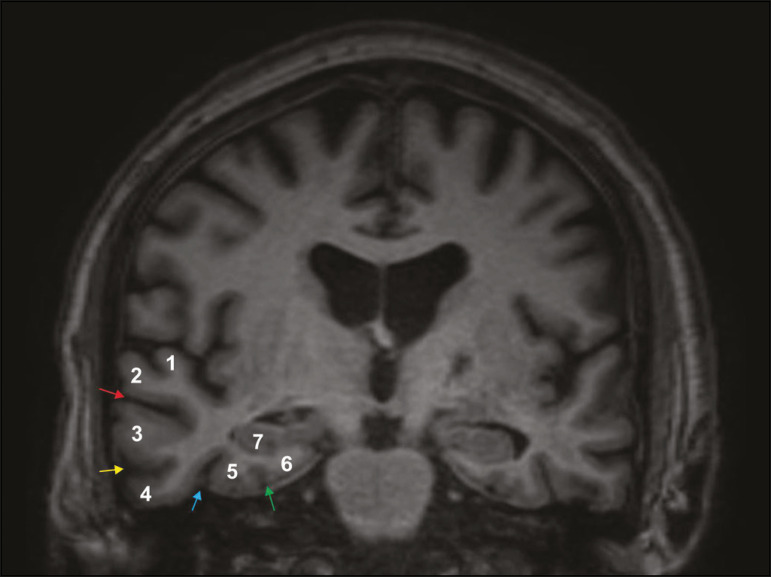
Coronal T1-weighted MRI sequence showing the normal anatomy of the temporal lobe. 1, transverse temporal gyrus; 2, superior temporal gyrus; 3, middle temporal gyrus; 4, inferior temporal gyrus; 5, lateral occipitotemporal gyrus; 6, medial occipitotemporal/parahippocampal gyrus; 7, hippocampus. Red arrow, superior temporal sulcus; yellow arrow, inferior temporal sulcus; blue arrow, occipitotemporal sulcus; green arrow, collateral sulcus.
